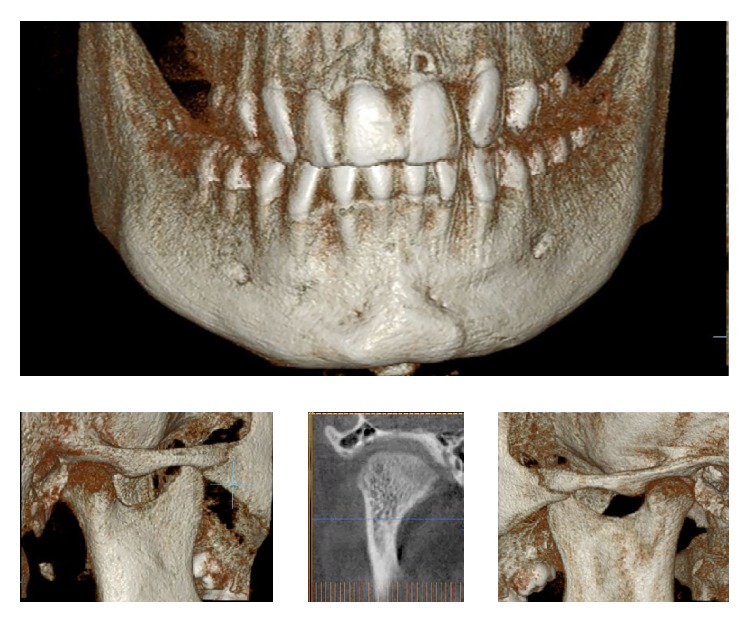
Cone beam CT views of the mandible after 18 months.
